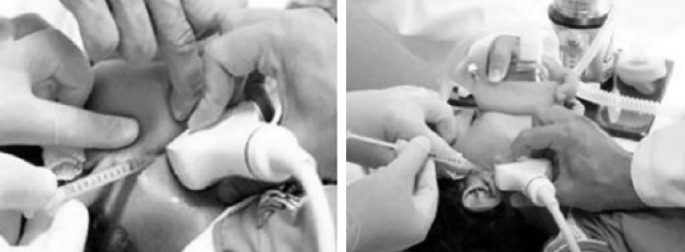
Direct ultrasound-guided botulinum toxin application in the parotid and submandibular glands, under sedation
